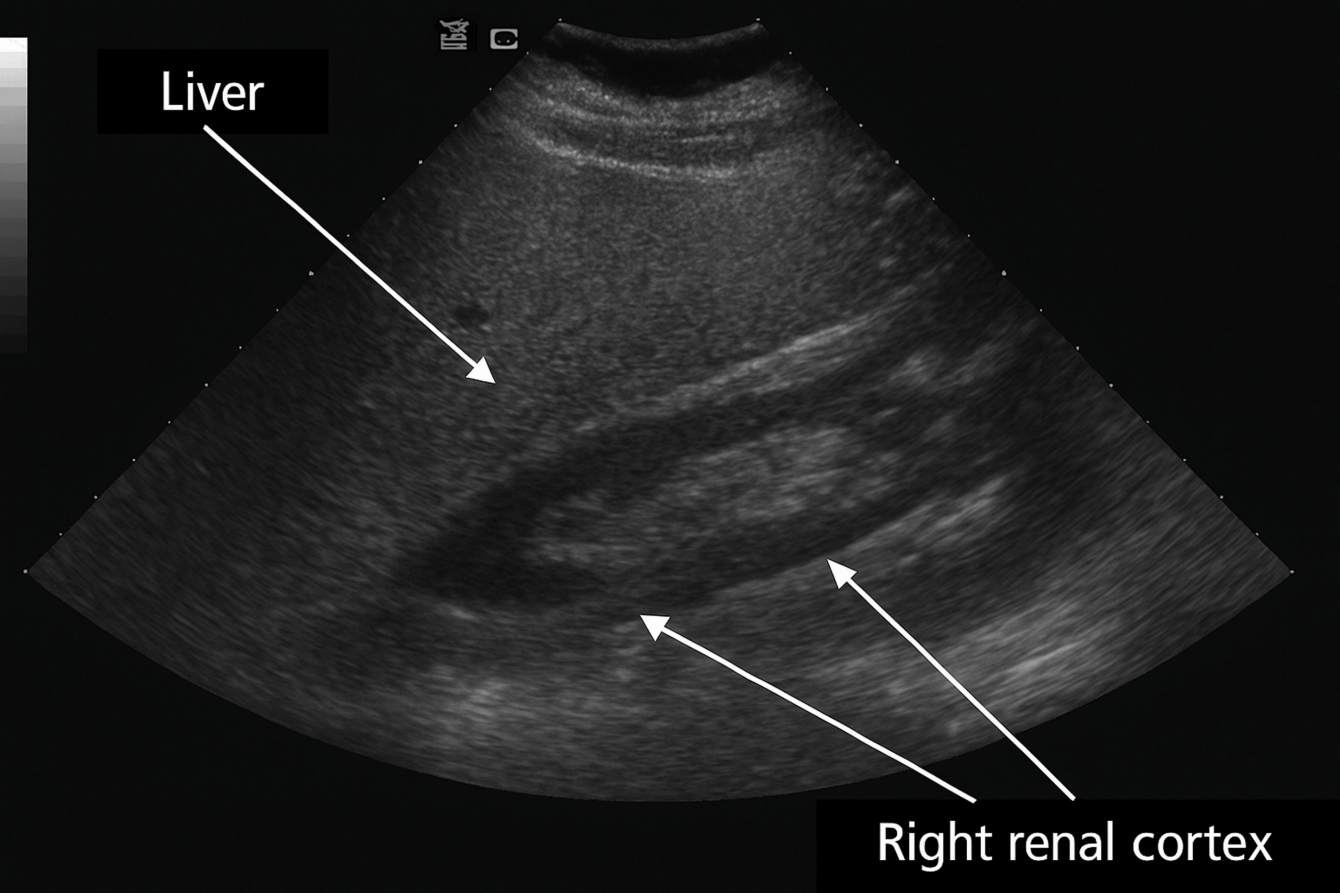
Abdominal ultrasound demonstrating hepatic steatosis Grayscale abdominal ultrasound image showing increased echogenicity of the liver parenchyma (labeled) compared to the adjacent right renal cortex, consistent with hepatic steatosis. The increased echogenicity obscures vascular markings, which is typical of fatty infiltration.
